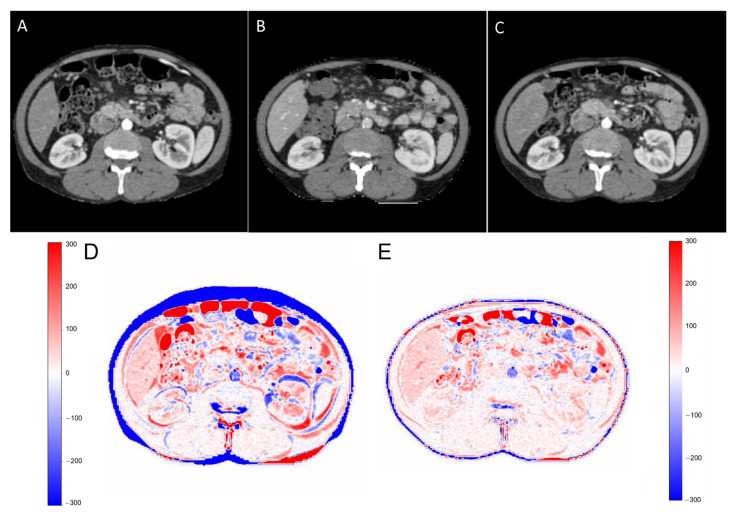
First row: The diagnostic CT (A), planning CT (B), and synthesized CT (C) at abdominal level/in abdominal window (40/400). Second row: (D) the HU intensity difference map between planning CT and diagnostic CT (pCT–dCT) and (E) between planning CT and synthesized CT (pCT–sCT). deepPERFECT’s predicted synthesized CT from diagnostic CT shows a high similarity to planning CT.
